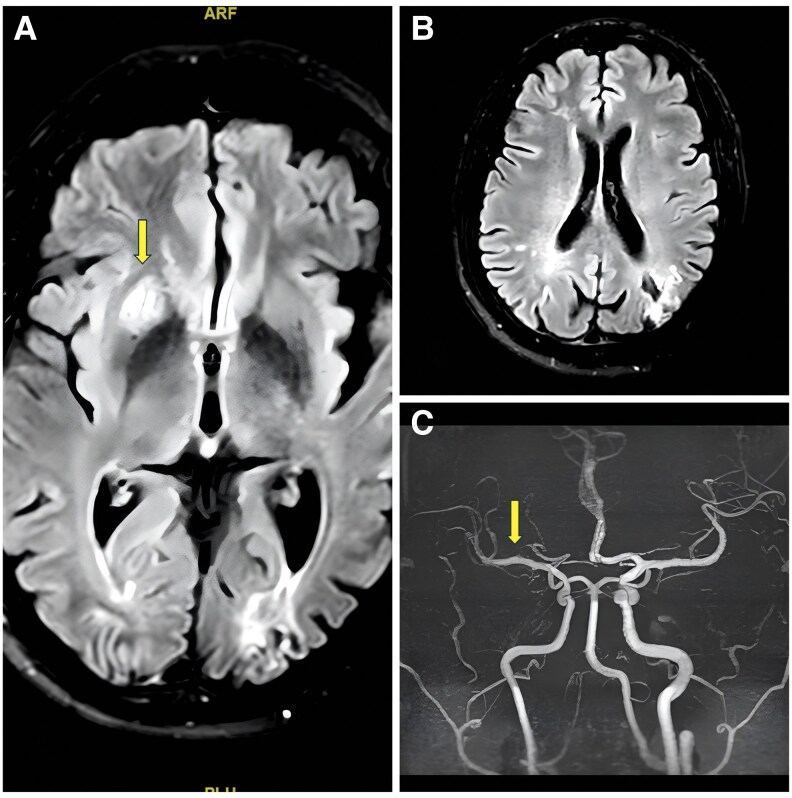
(*A*) Magnetic resonance imaging of the brain with FLAIR images of the patient showing hyperintensity in right-sided caudate and putamen suggestive of an acute infarct (shown by long arrow). (*B*) The magnetic resonance imaging of the brain of the patient also shows a bilateral old parieto-occipital infarct with gliosis. (*C*) Magnetic resonance angiography of the patient showing right-sided paucity of M3 branches of middle cerebral artery (MCA) (shown by long arrow).
